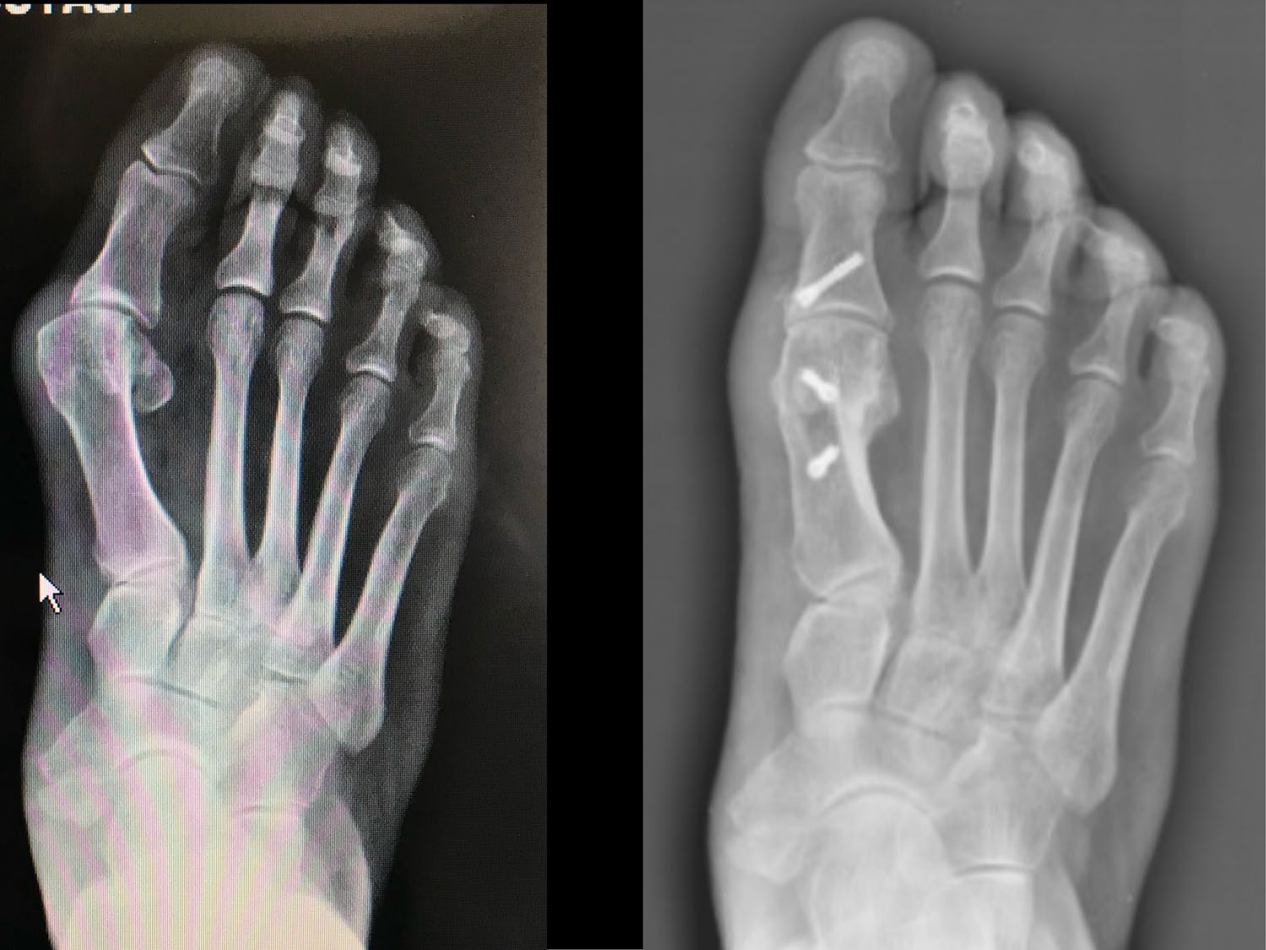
Example of a preoperative (left) and postoperative (right) weightbearing radiographic dorsoplantar view in a patient enrolled in this series. Two screws were used to fix the Scarf osteotomy and one (only case in the series) for the Akin osteotomy. Sesamoid position improved from 6 to 2 according to the Hardy and Clapham classification system. No lateral soft tissue release was performed
